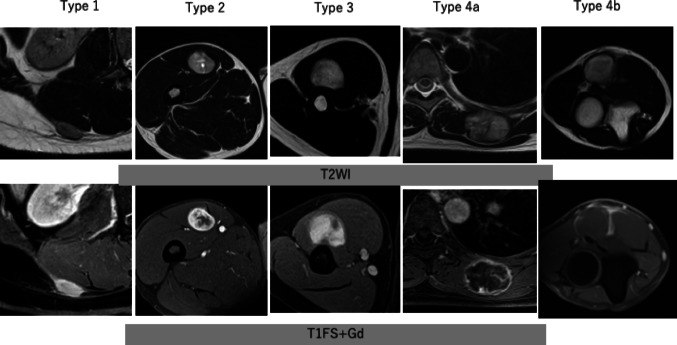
MRI images of a case reflecting the four type classifications. The upper panel is the T2-weighted axial image, and the lower panel is a post-contrast fat suppressed T1-weighted axial image. Type 1 shows entire tumor enhancement ; type 2 shows non-enhancing area only in the center; type 3 shows non-enhancing area including outside the center; and type 4a shows a predominantly non-enhancing area with minimal peripheral enhancement. Type 4b shows a completely non-enhancing area with perilesional enhancement
